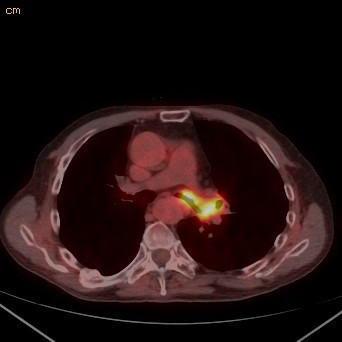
PET-CT (positron emission tomography - computer tomography) scan showing FDG-avid circumferential thickening of distal LMS bronchus and left upper lobe (SUV of 7.4).
